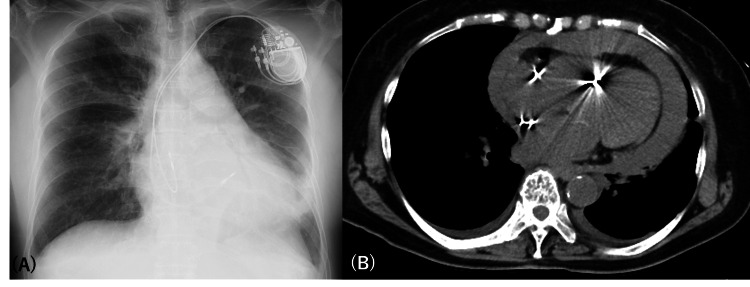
(A) Chest X-rays at the first visit to the previous physician. (B) The axial view of CT of the chest showed moderate pericardial effusions and slightly bilateral pleural effusions at the first visit to the previous physician
